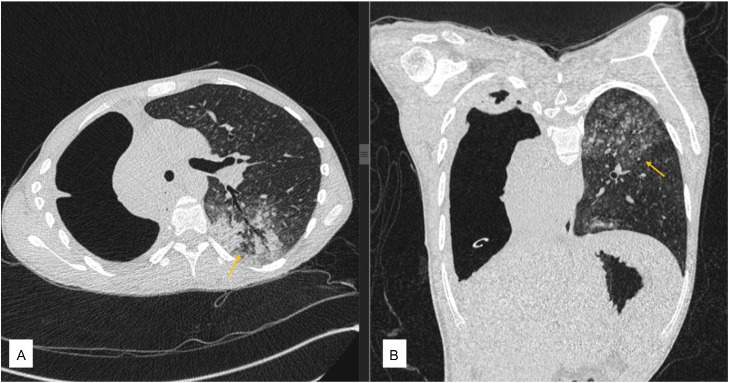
Chest computed tomography lung window axial view (A) and coronal view (B) revealed centrilobular nodules “tree-in-bud” surrounded with ground-glass opacity and patchy consolidation in the left lung (orange arrow).
